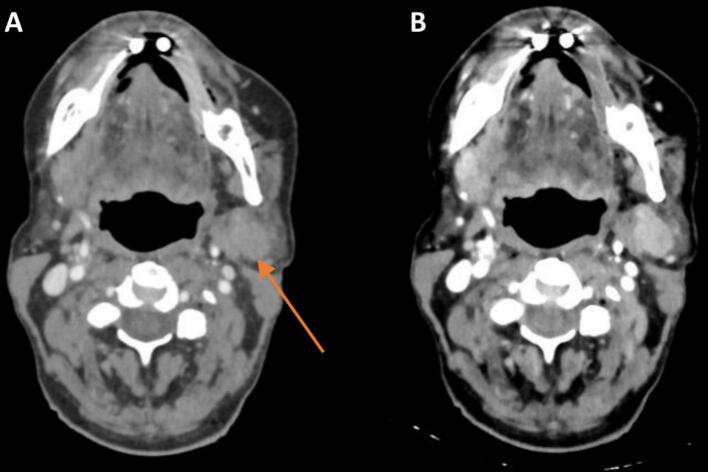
Example of the enhanced iodine contrast at low keV virtual mono-energetic images. *A) Virtual mono-energetic image at 70 keV, which is equivalent to a conventional CT image acquired at 120 kV (typically used for RT planning). Arrow indicates the lesion. B) Virtual mono-energetic image at 40 keV with improved iodine contrast in the tumor, which may lead to a more reliable tumor delineation, in this case especially at the medial border. Both images are displayed at window width 400 and window level 40.*
